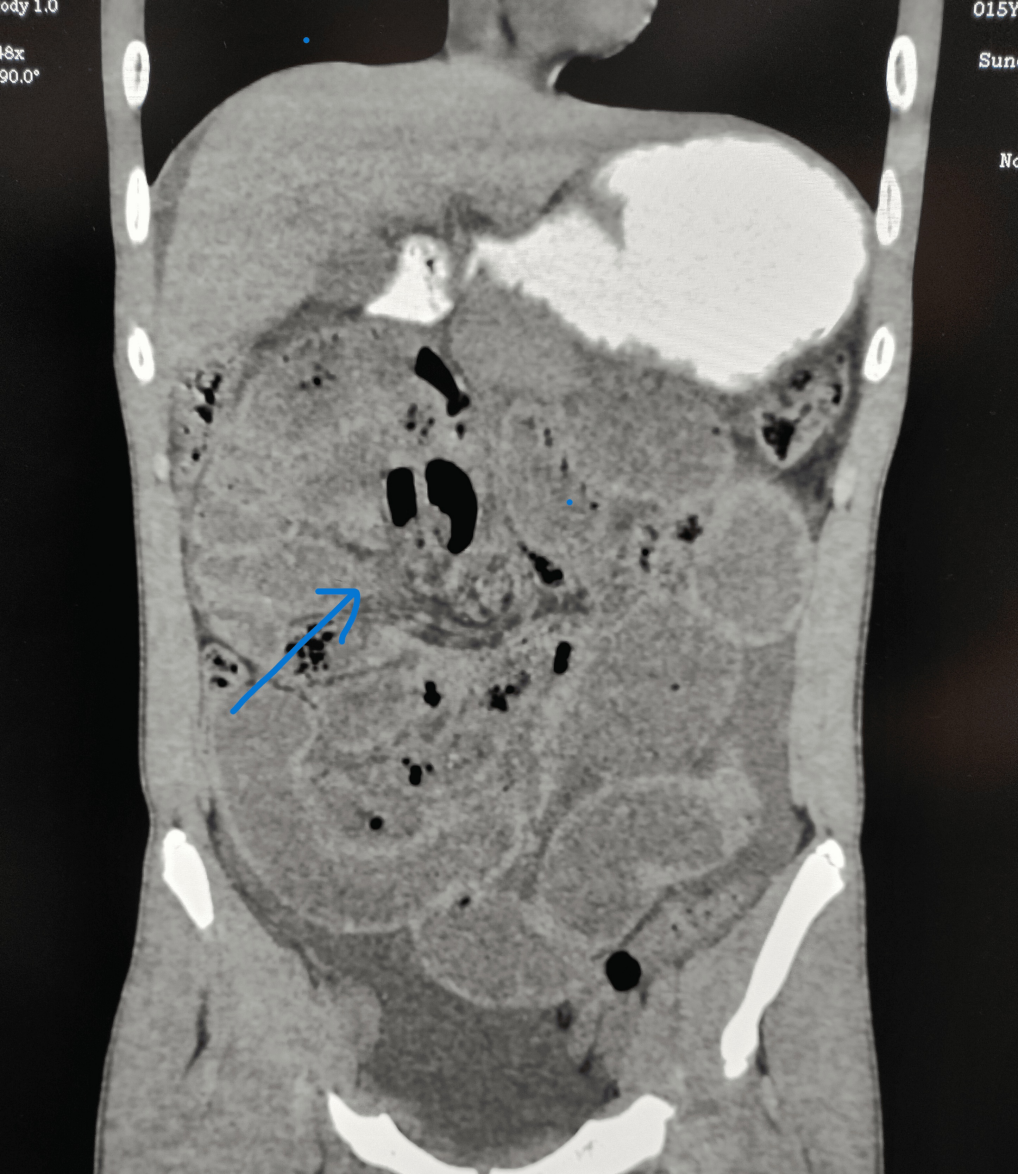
Plain computed tomography scan of abdomen and pelvis. The scan shows a sac-like structure in the right upper quadrant with entrapment of small bowel loops and twisting of vessels at the neck of the sac along with displacement of the duodenum (blue arrow).
